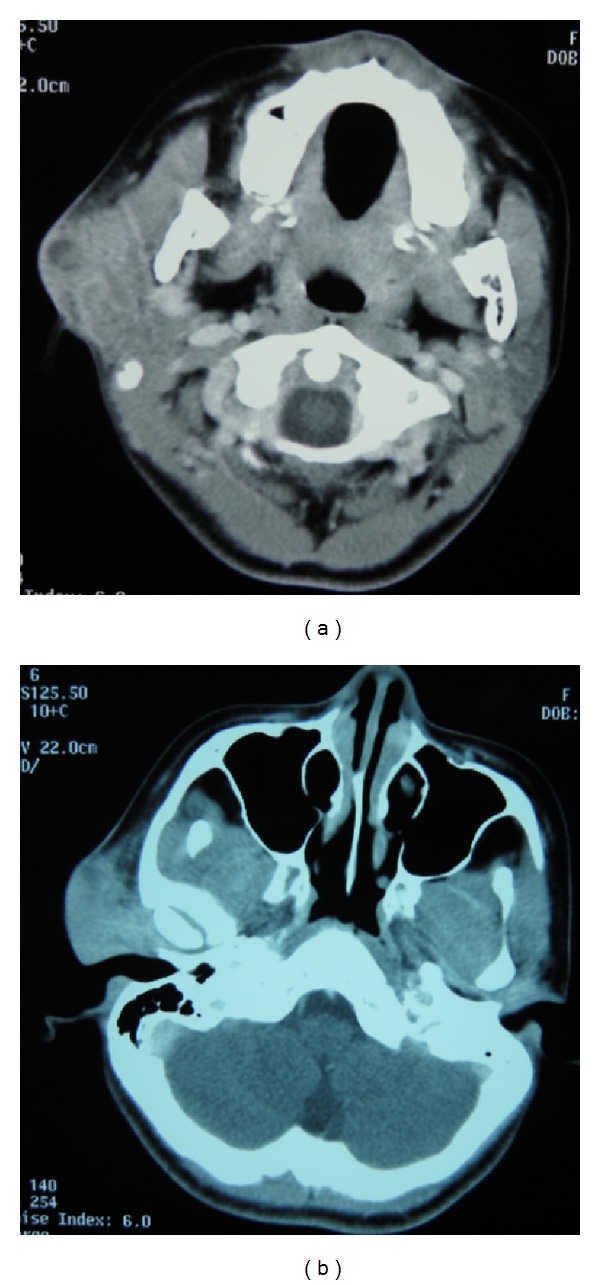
CT findings. (a) A 45 × 40 mm tumor is apparent in the right parotid gland. (b) Infiltration into surrounding tissues is evident, including skin, anterior wall of the external auditory meatus, masseter muscle, and temporomandibular joint.
